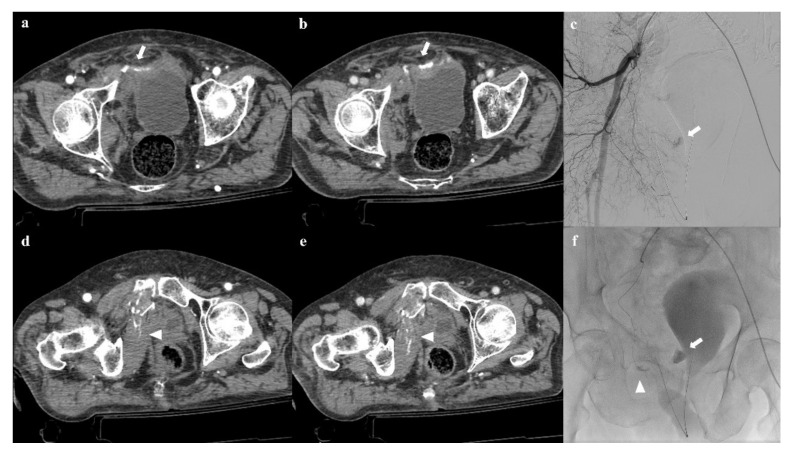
Pelvic ring trauma, characterized by fracture of right superior and inferior pubic rami. Axial CT image in the arterial phase shows active extravasation of contrast medium near the right superior pubic branch (arrow in (a)), which increases in the venous phase of the examination (arrow in (b)). Similar active extravasation is documented near the internal obturator muscle (arrowheads in (d,e)). Selective angiography confirmed the two blood extravasation spots, with origin from branches of the right obturator artery (arrows and arrowhead in (c,f)); both the bleeding spots were optimally embolized.
